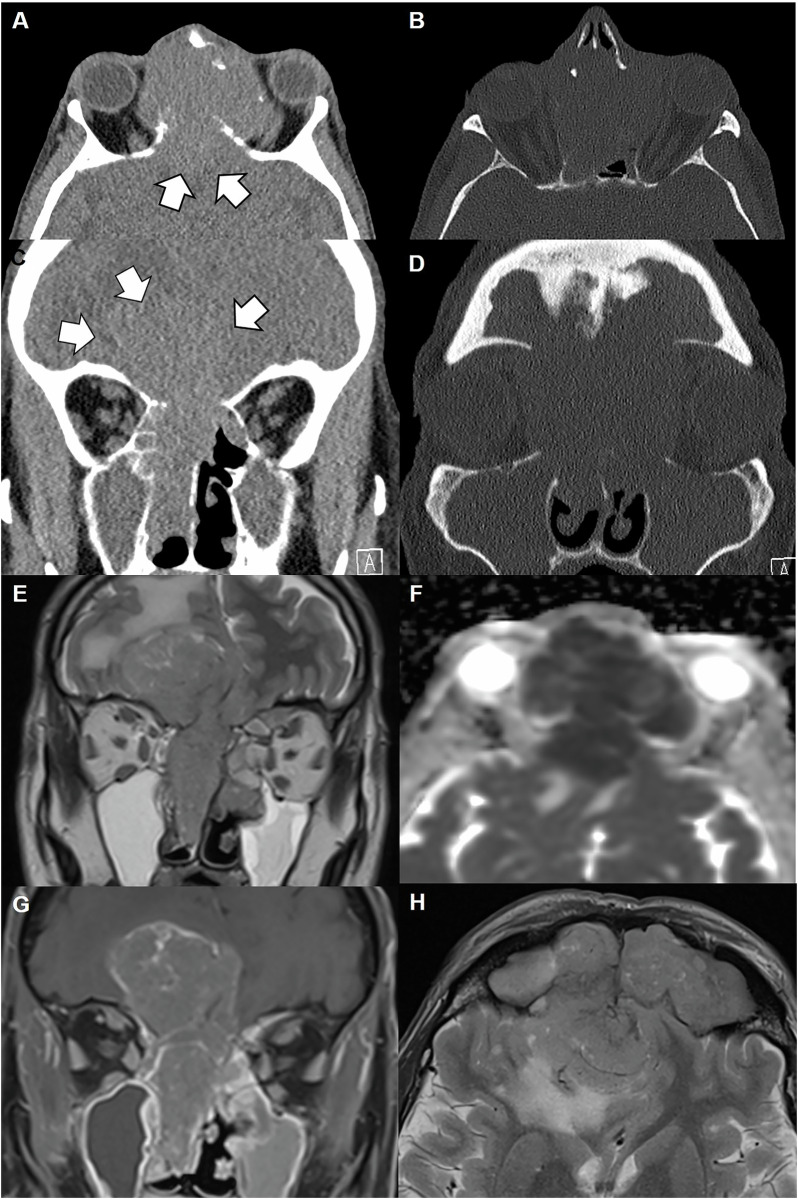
Undifferentiated sinonasal squamous cell carcinoma (SCC). Axial (A) and coronal (C) soft tissue window and axial (B) and coronal (D) bone window CT images. Coronal T2w (E), axial ADC (F), coronal T1w post contrast (G) and axial T2w (H) MR images. There is a large inhomogeneous mass centered in the right nasal cavity with extension through the destructed ethmoid air cells and the lateral orbital walls into the orbits. There is extension through the destructed cribriform plate/planum sphenoidale into the anterior cranial fossa, giving the lesion a “dumbbell” or “waist” like appearance. The very low ADC values indicate high cellularity. There is vasogenic edema of the adjacent frontal lobe
